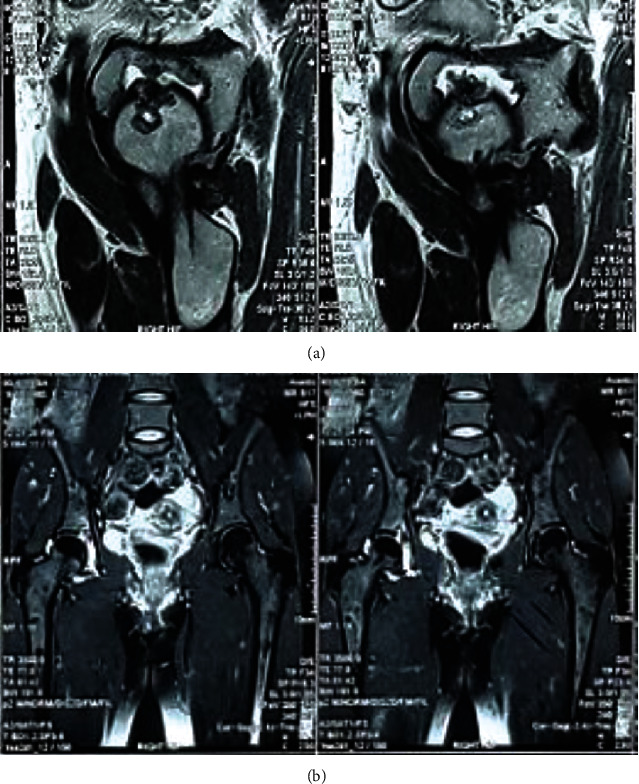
Synovium appears as hyposignal on T2-wieghted preoperative MRI in the sagittal plane and enhances after the gadolinium injection (a). Preoperative MRI showing the characteristic synovial process with low-signal intensity in fat-suppressed T2-weighted sequences (b).
